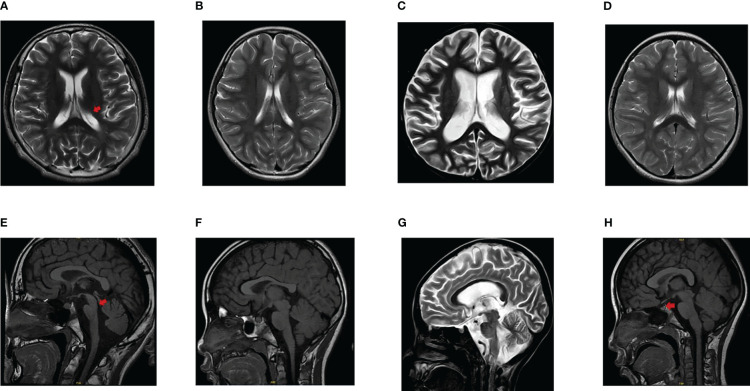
Findings of brain MRI in patients. (A, E) The ventricular system was enlarged (arrowhead in A), the sulci and cisterns were widened, the brain stem morphology was poor (arrowhead in E), and lacked a clear high signal indicating the neurohypophysis in patient 1. (B, F) The MRI finding presented as an abnormally small neurohypophysis without encephalatrophy in patient 2. (C, G) Atrophic changes of the brain was found and lacked a clear high signal indicating the neurohypophysis in patient 5. (D, H) No signs of brain atrophy and a normal high signal of neurohypophysis (arrowhead in H) were displayed in patient 6.
